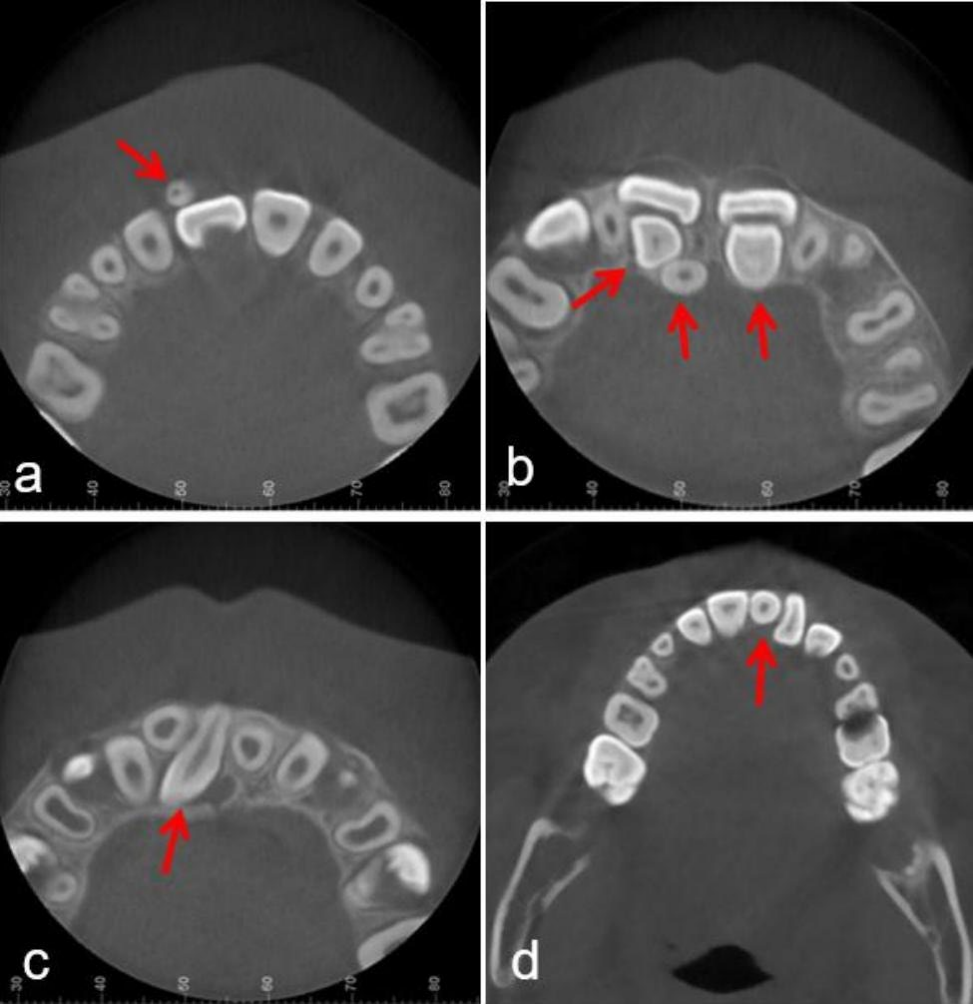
Representative CBCT images exhibiting diverse position of ST. (a) labial of the arch; (b) palatal of the arch; (c) throughout the arch; (d) median of the arch. The red arrows point to the ST in the CBCT images
